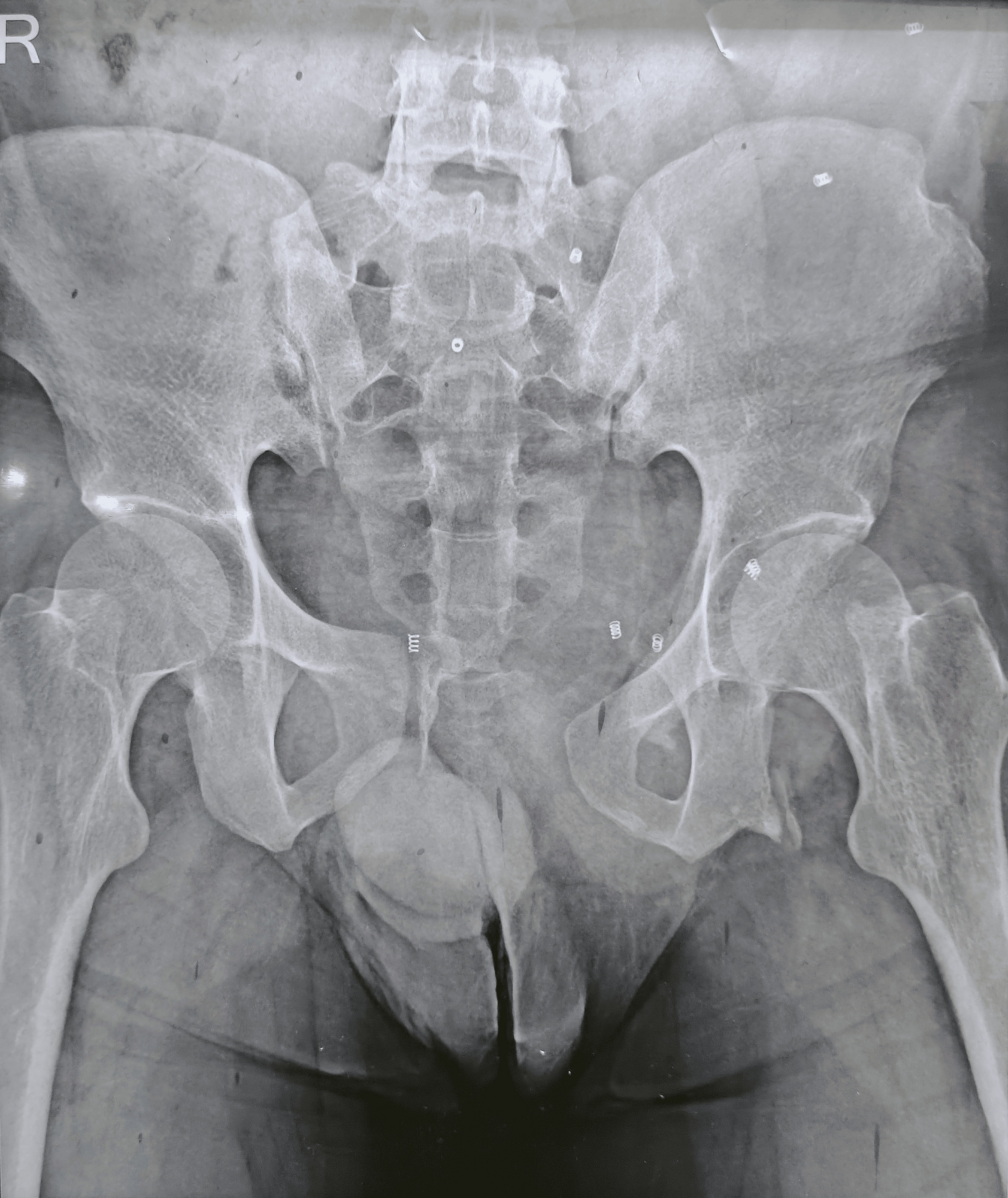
Plain pelvic radiograph demonstrating widening of the pubic symphysis
